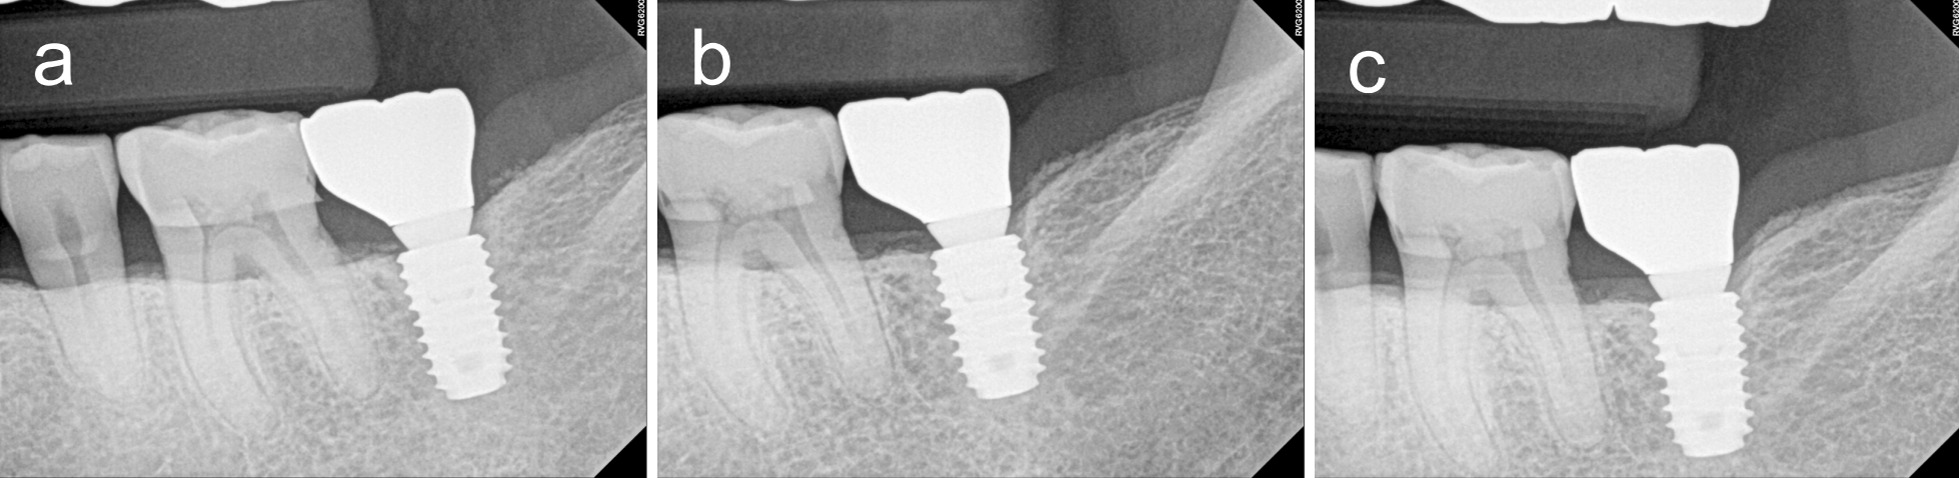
Periapical radiographs of a dental implant. a Immediately after loading; b 1 year after loading; c most recently
